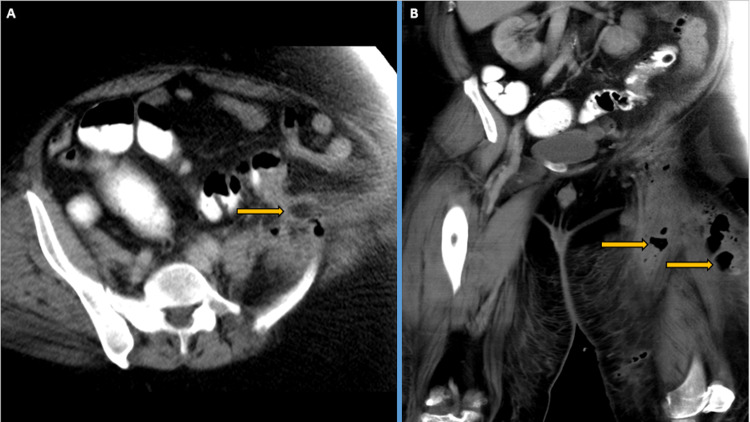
Day eight CT (A) Axial contrast-enhanced computerised tomography (CECT) pelvis image showing persistence of the fistulous tract from the left-sided descending colon extending to the left-sided retroperitoneum overlying the left iliopsoas. (B) Coronal CECT image showing interval regression of the liquified thigh abscess with residual few air pockets in the left proximal thigh, marked with arrows.
